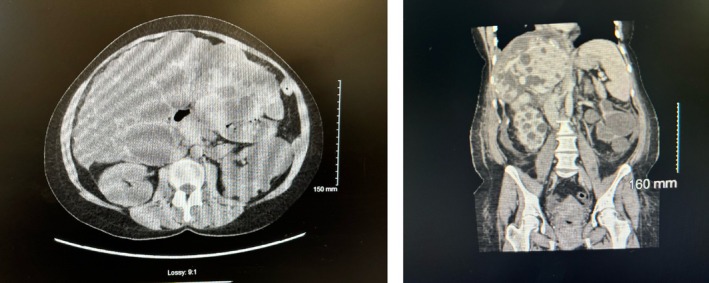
CT Scan images of the proband showing liver and kidney cysts.
